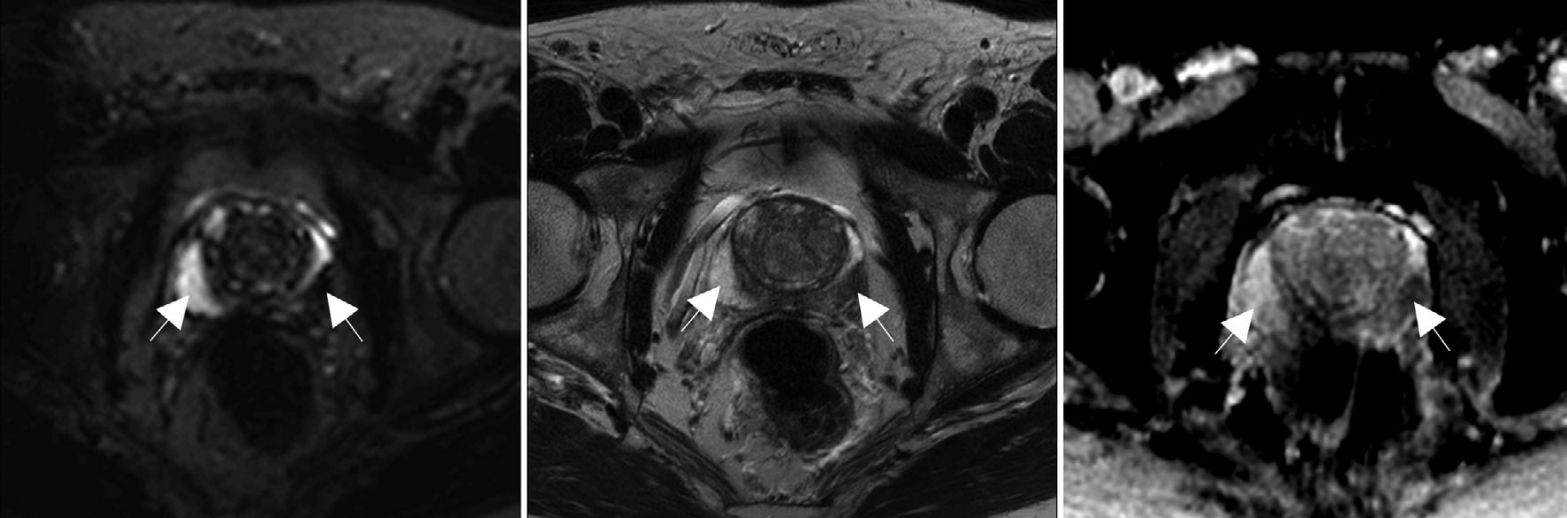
LWF map, axial T_2_ image, and ADC map for one patient. The region of healthy tissue is highlighted by the arrow on the left of each image, the tumor by the arrow on the right of each image. This figure shows distortions in the PZ in the diffusion‐weighted image, highlighting a disadvantage of DWI over ME‐T_2_ modeling.
